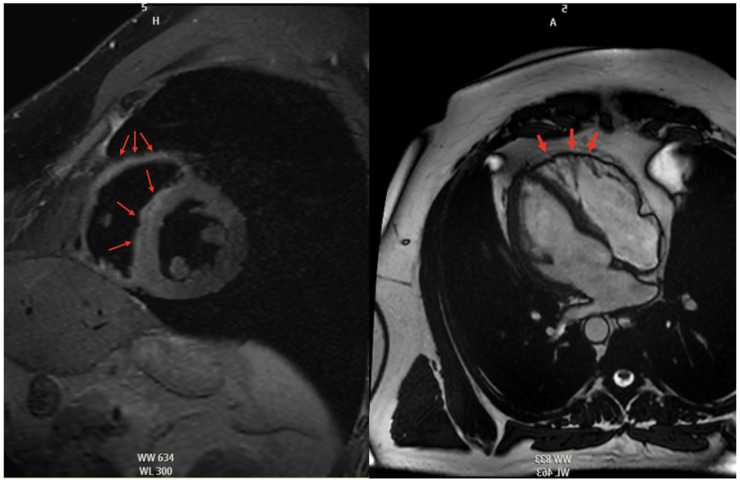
Cardiac Magnetic Resonance Images (MRI) showcasing characteristic fibrofatty infiltration of the right ventricular myocardium (red arrows). (Left) Fat-suppressed contrast-enhanced T1-weighted MRI. (Right) Steady-state free precession (SSFP) MRI.
